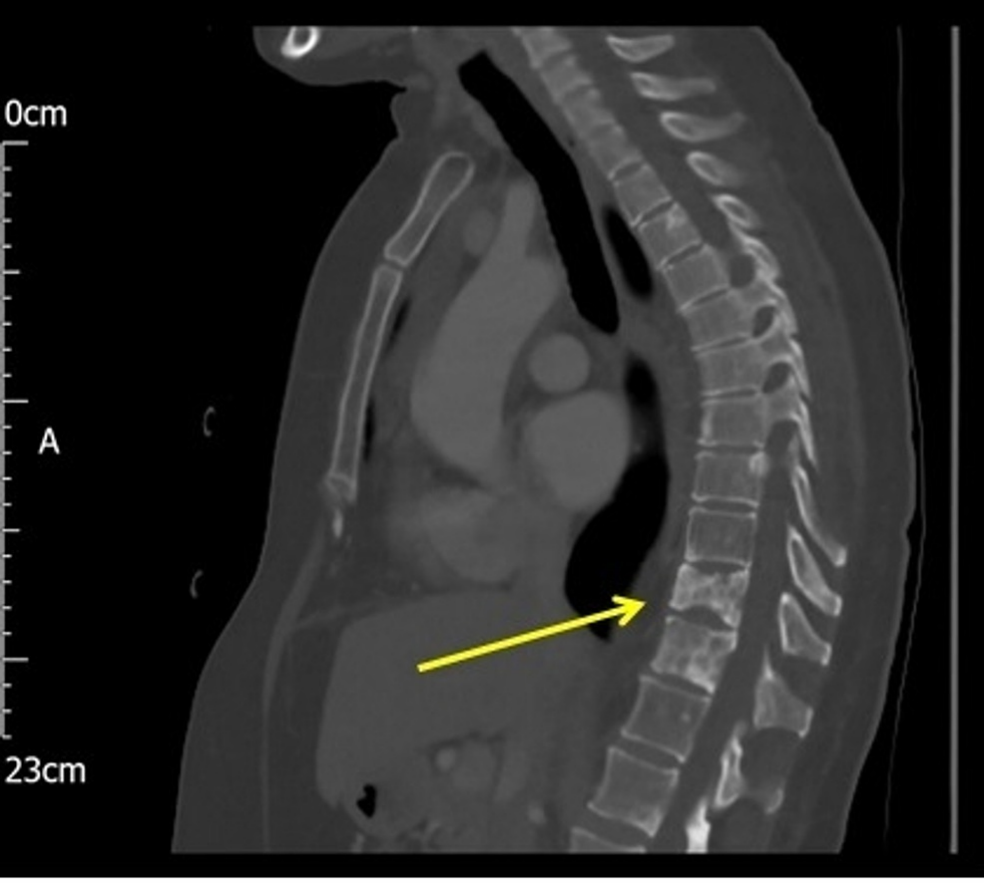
CT chest: sagittal view with multiple lytic and sclerotic lesions in the thoracic spine (yellow arrows). CT: computed tomography
